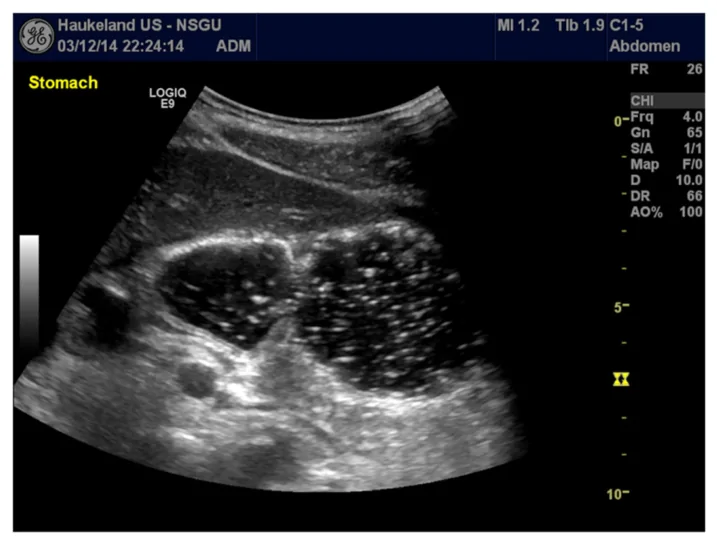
A 2D ultrasonographic image showing an antral contraction. Ultrasound is a real-time imaging modality with high temporal and spatial resolution, making it particularly well suited for assessing gastric motility.
